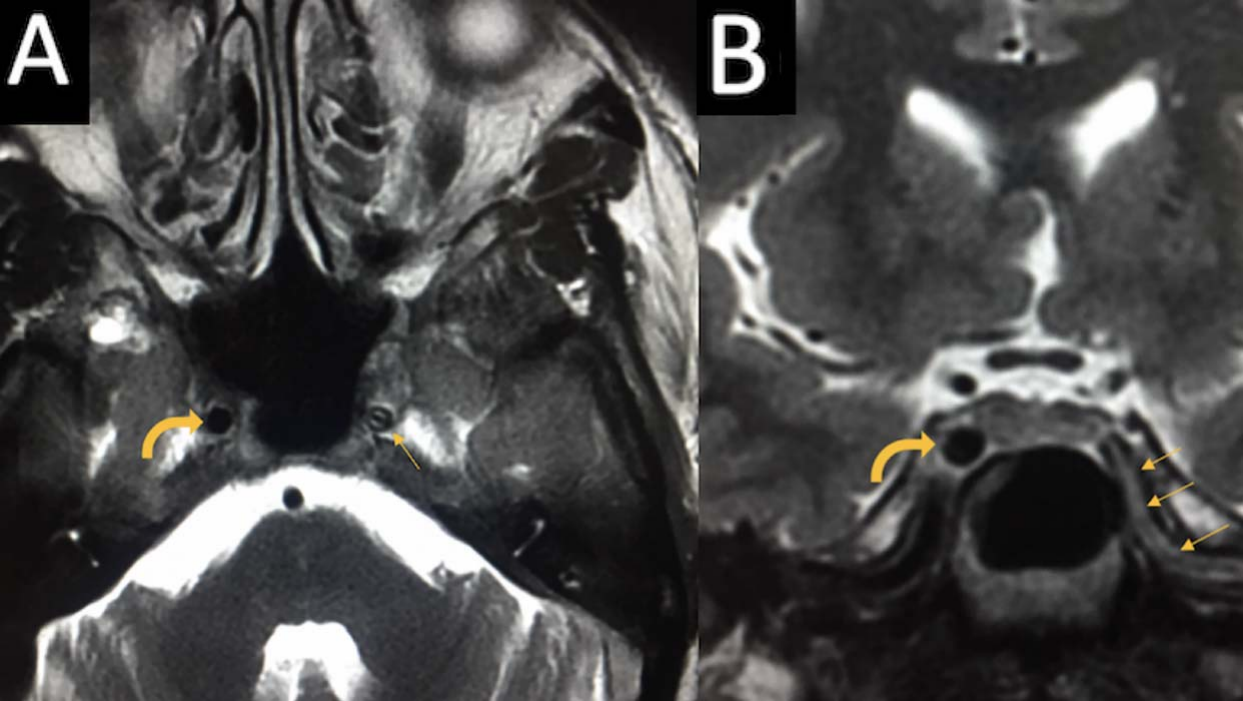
Images A and B are axial and coronal views of the T2 MRI sequence of the brain, respectively. Image A shows that the petrous segment of left ICA (straight arrow) is abnormal with a thin central hypointense signal (black lumen) which is compressed with a crescent of high signal (haematoma) in the vessel wall. In comparison, the right ICA shows a normal signal void (curved arrow). Image B shows that the petrous segment of the left ICA (straight arrows) shows abnormal vessel contour in continuity with a thin linear central hypointensity (black lumen) which is compressed with a high signal (haematoma). In comparison the right ICA shows normal vessel calibre (curved arrow).
